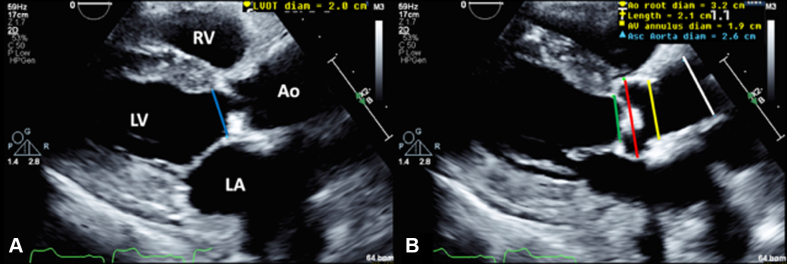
LVOT and Aortic Root Measurements Parasternal long-axis view measurements of the left ventricular outflow tract diameter in mid-systole (left, blue line), aortic annulus diameter (right, green line), sinus of Valsalva diameter (right, red line), ST-segment junction diameter (right, yellow line), and ascending aorta diameter (right, white line). Abbreviations as in Figure 2.
